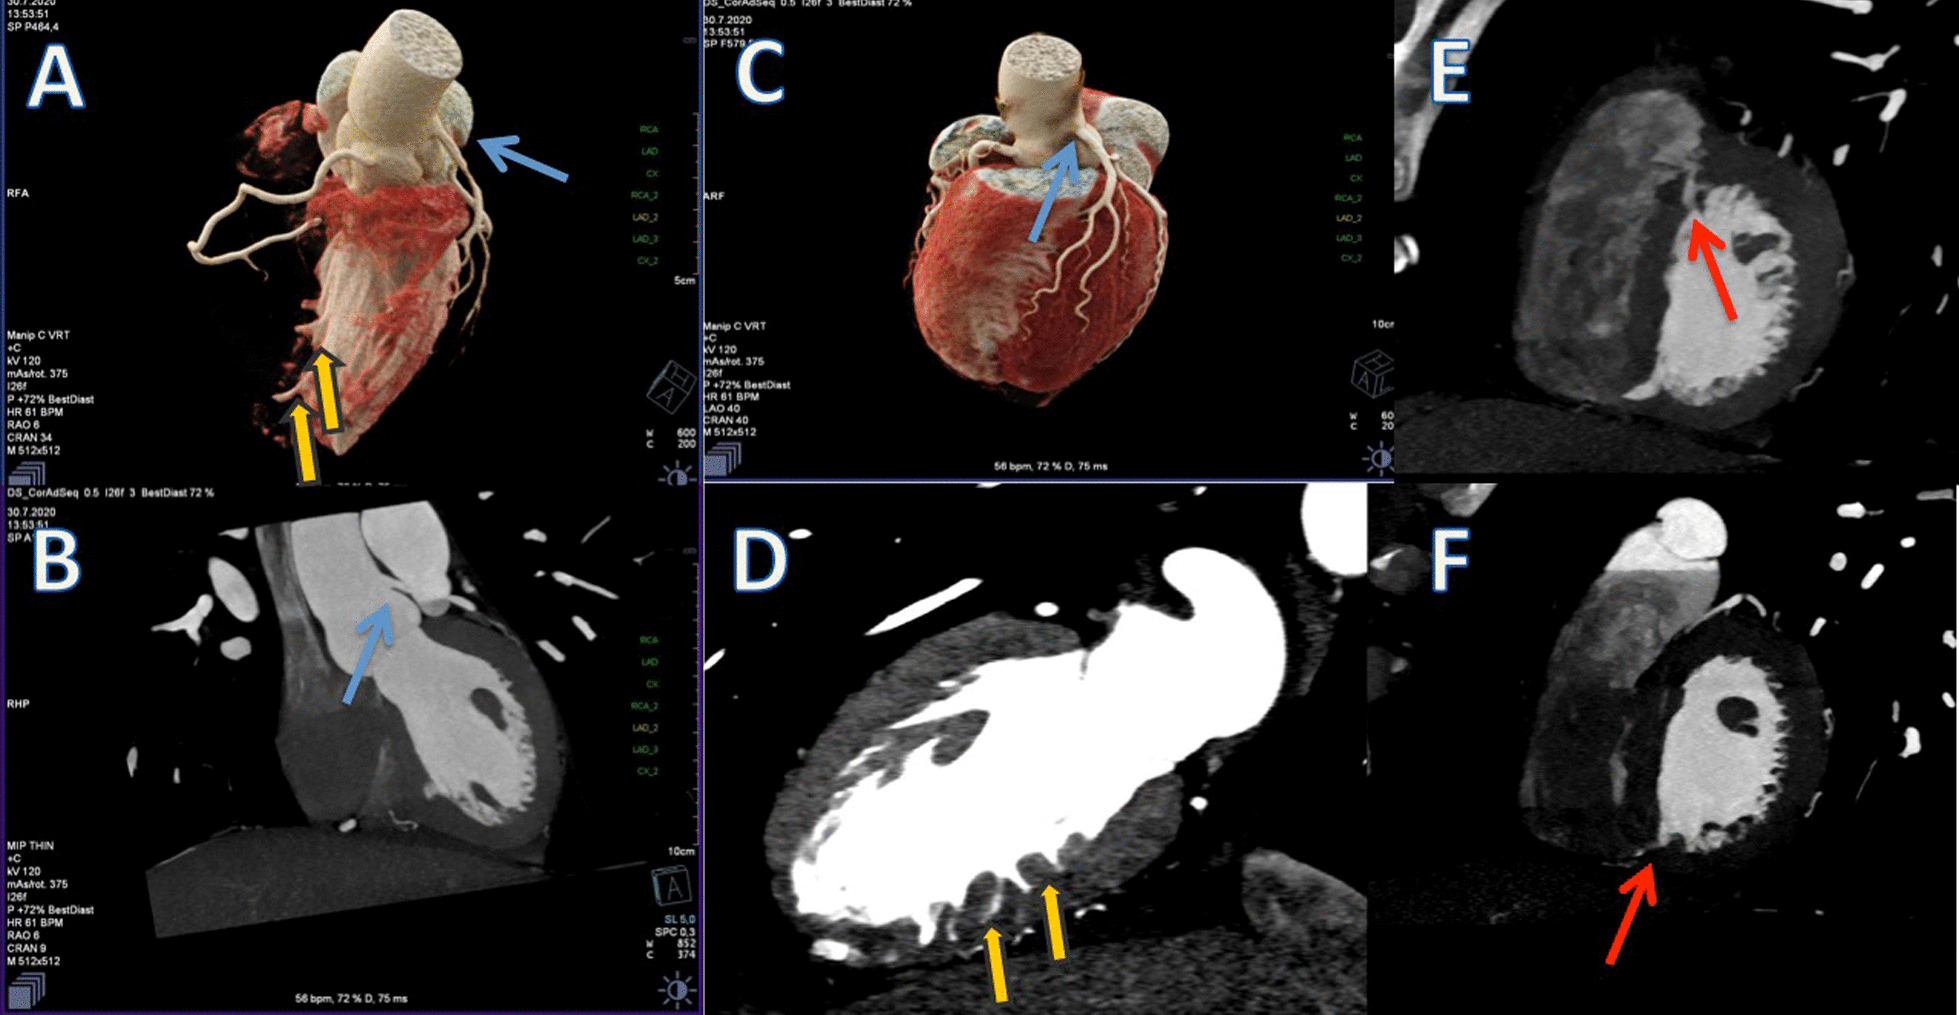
A Cardiac CT 3D volume rendering image demonstrates the high take-off LMCA(blue arrow) and multiple crypts in the interventricular septum(yellow arrows). B Cardiac CT demonstrates high take-off LMCA(blue arrow). C Cardiac CT 3D volume rendering image demonstrates the high take-off LMCA from another view. D Cardiac CT 2 chamber view demonstrates the multiple crypts in the inferior wall of the left ventricle(yellow arrows). E Cardiac CT short axis view shows the deep crypt in the anterior left–right ventricle insertion point(red arrow). F Cardiac CT short axis view shows the deep crypt in the inferior left–right ventricle insertion point(red arrow)
